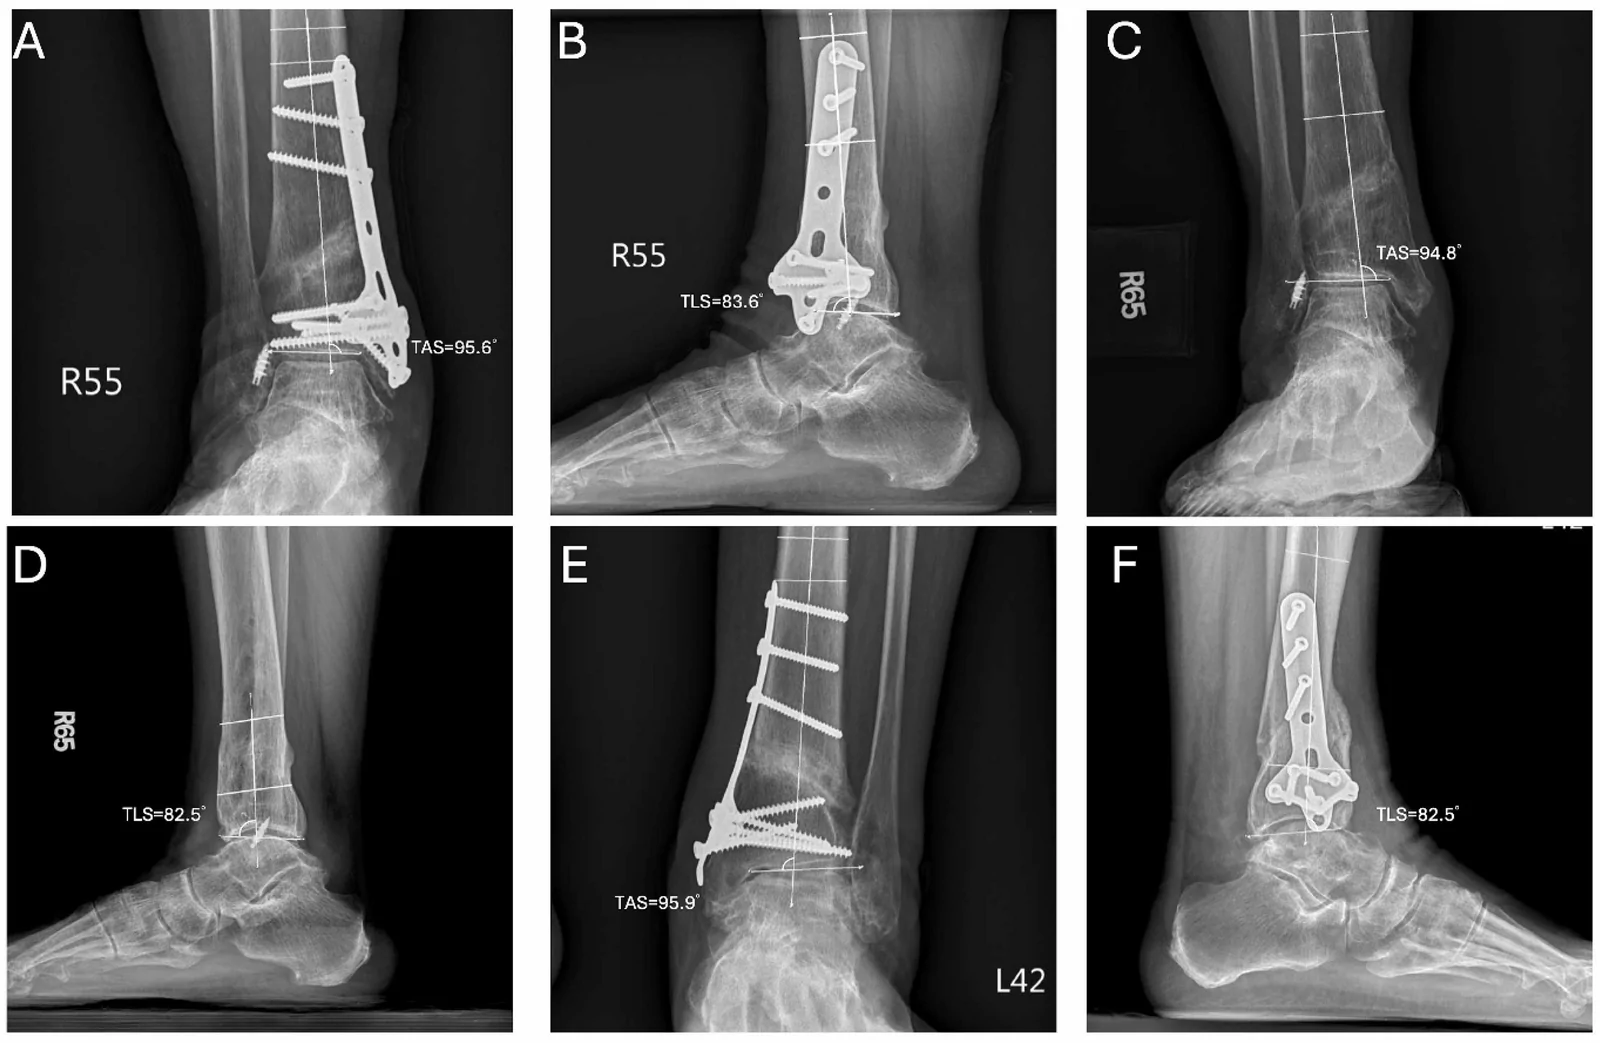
Postoperative weight-bearing radiographs following DTOO. (A) (Stage IIIa) Postoperative AP radiograph at 1 year after DTOO with strut allograft insertion and medial plating, ATFL repair additionally. Correction of joint alignment is noted with restoration of the joint line and reduction in the tibiotalar tilt. Allograft incorporation and bony union are observed without hardware failure. TAS 95.6°, MMA 27.7°, TTA 3.9°, TTS 92.3°. (B) (Stage IIIa) Postoperative lateral radiograph at 1 year. The anterior impingement spur and dome flattening observed preoperatively have been addressed via realignment. TLS improved to 83.6°, and sagittal plane congruency of the tibiotalar joint is preserved. (C) (Stage IIIa) Postoperative AP radiograph at 3 years, 1 year after plate removal. Joint space remains preserved with no recurrence of varus tilt or medial gutter narrowing. The allograft appears fully consolidated without subsidence. No signs of osteophyte formation or sclerosis. TAS 94.8°. (D) (Stage IIIa) Postoperative lateral view at 3 years. Sagittal alignment is maintained, with no anterior impingement recurrence. The tibial plafond and talar dome show no progression of arthritic changes. TLS 82.5°. (E) (Stage IIIb) Postoperative AP radiograph at 1 year after DTOO with strut allograft insertion and medial plating, ATFL repair additionally. Correction of joint alignment is noted with restoration of the joint line and reduction in the tibiotalar tilt. Allograft incorporation and bony union are observed without hardware failure. TAS 95.9°, MMA 36.3°, TTA 1.4°, TTS 89.3°. (F) (Stage IIIb) Postoperative lateral radiograph at 1 year. The anterior impingement spur and dome flattening observed preoperatively have been addressed via realignment. TLS improved to 82.5°, and sagittal plane congruency of the tibiotalar joint is preserved.
